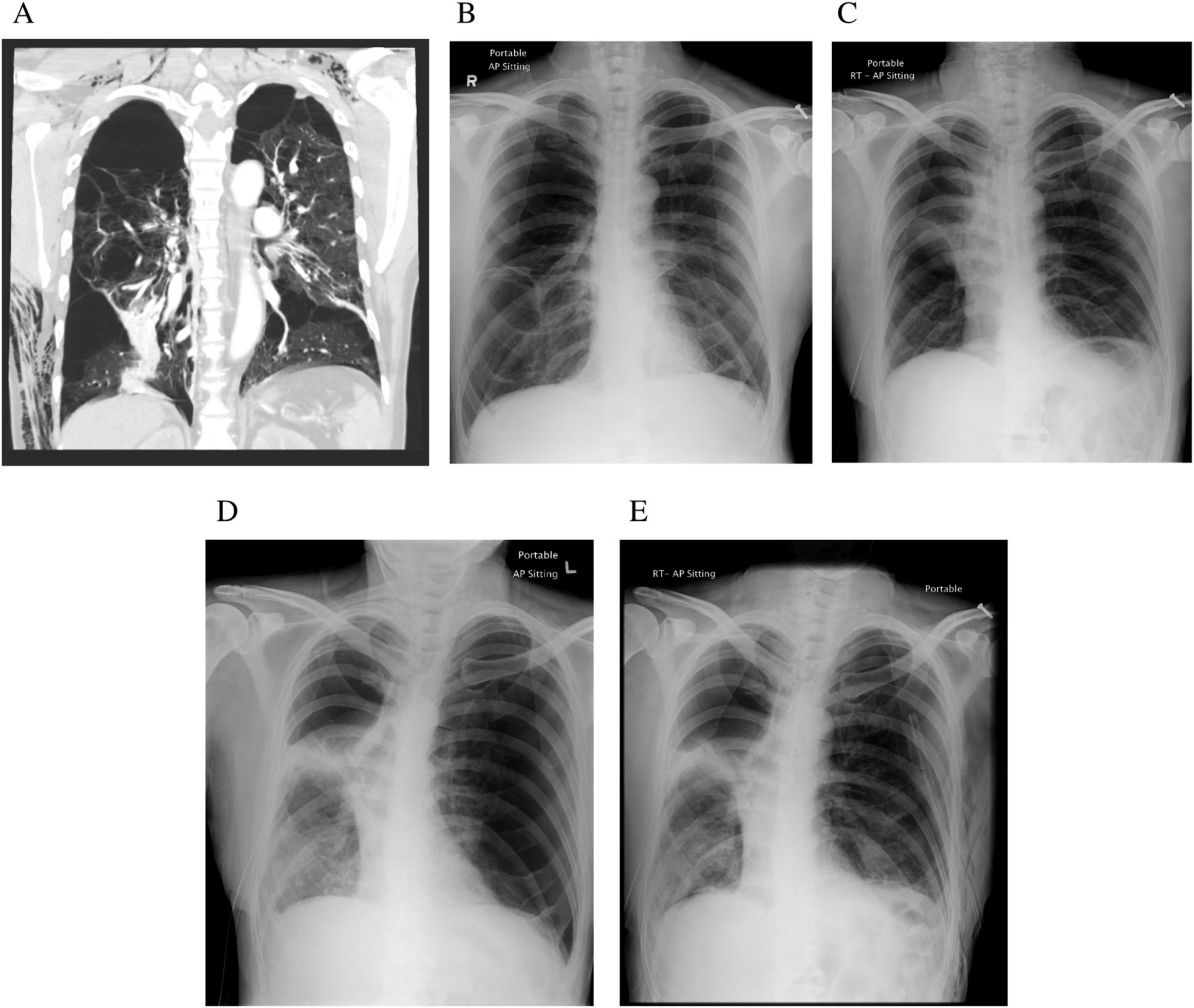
(A) Coronal view of computed tomography (CT) thorax. (B) Chest radiograph (CXR) immediately before endobronchial one‐way valve (EBV) insertion. (C) CXR immediately after EBV insertion. (D) CXR showing new left pneumothorax. (E) CXR showing chest tube inserted for left pneumothorax.
